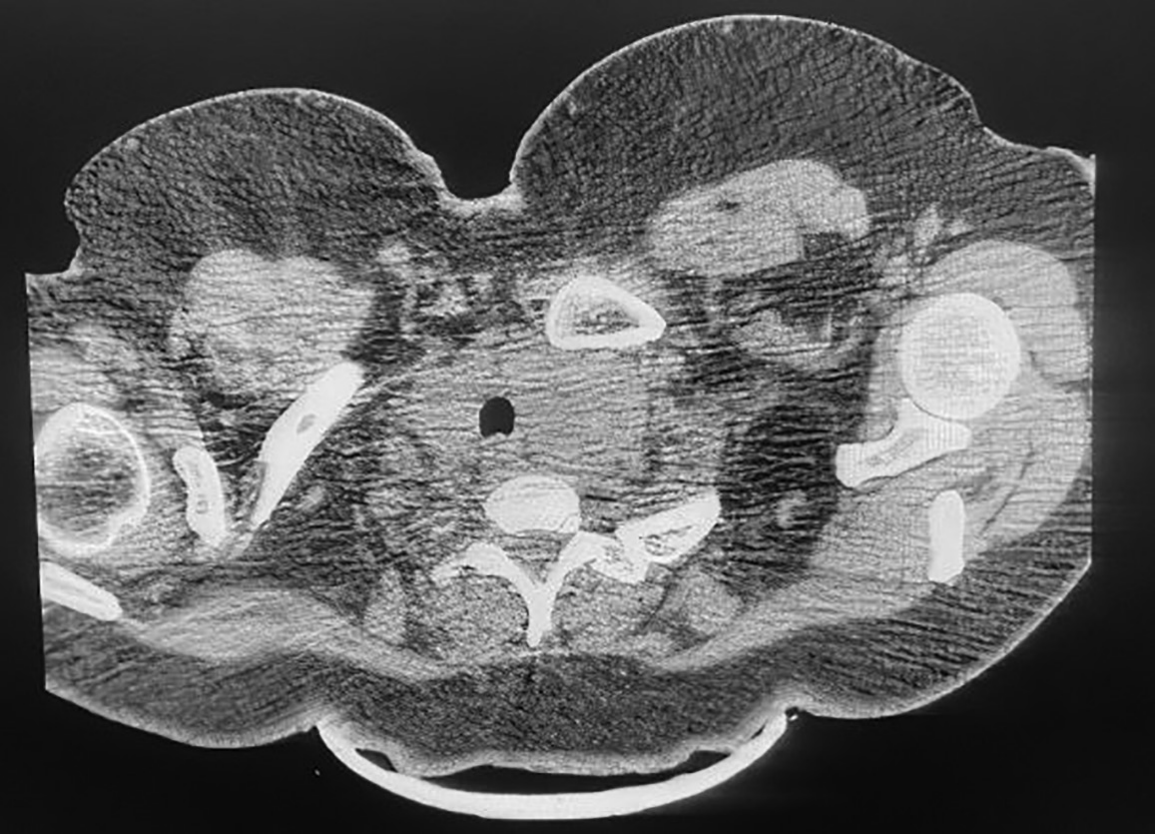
Computed tomography (CT) scan of the neck showing a large retrosternal goiter (RSG).
